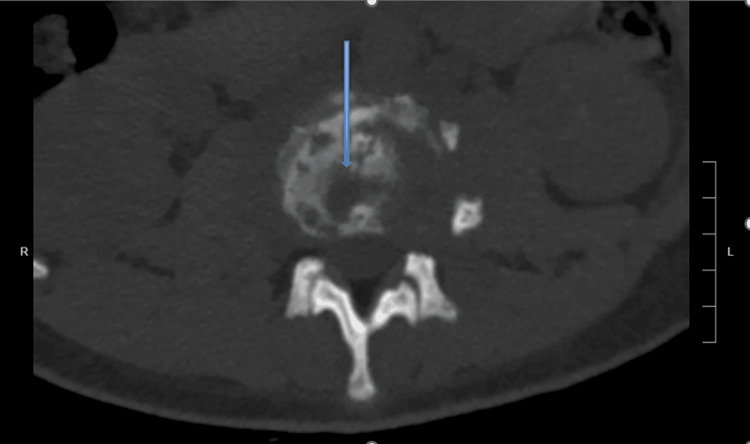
CT of the lumbar spine without contrast showing extensive erosive bony changes at the L2-L3 levels, with loss of vertebral body height consistent with known discitis osteomyelitis. There is severe left and mild to moderate right neural foraminal narrowing at the L2-L3 levels. CT: computed tomography
